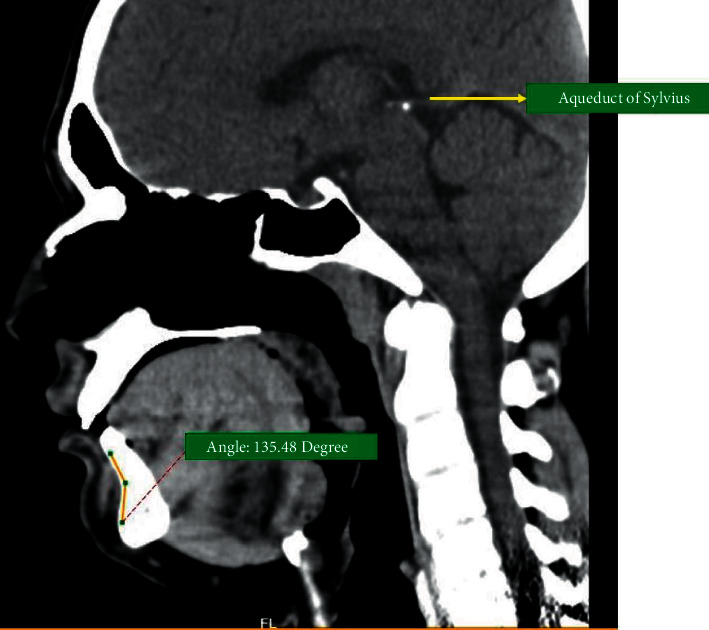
Chin angle in a 2D CT scan of a noncondylar fracture patient.
